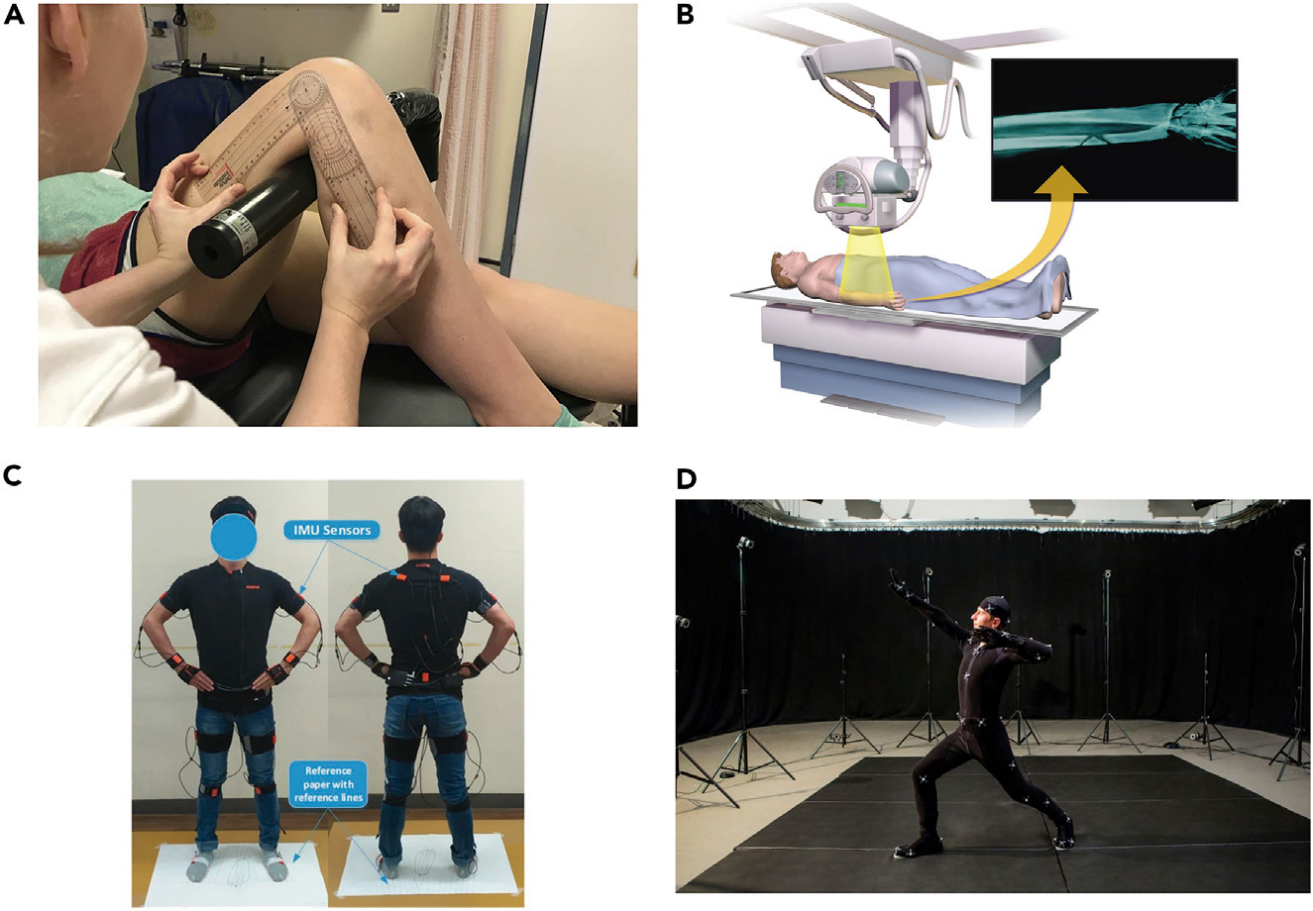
Several representative posture assessment methods from previous studies (A) Goniometry.^12^ (B) Radiography.^36^ (C) Inertial sensor based Xsens MVN.^24^ (D) Optical motion capture system.^37^
